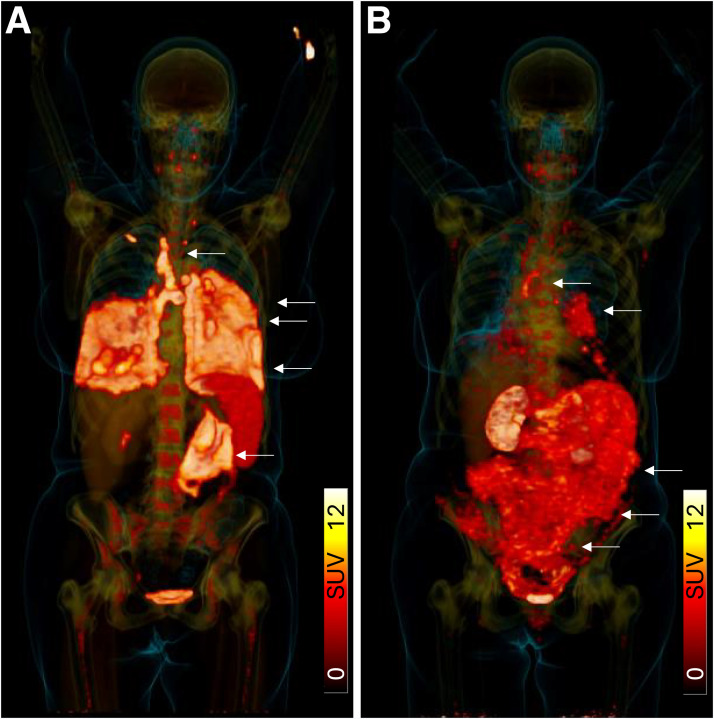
CXCR4-directed RPT with [^90^Y]Y-Pentixather holds promise particularly for salvage treatment of leukemias and lymphomas. In a 65-y-old woman with T-cell prolymphocytic leukemia and progressive disease after 4 lines of therapy, salvage treatment with [^90^Y]Y-Pentixather was offered, followed by autologous stem-cell transplantation. No further cancer drug therapy was performed. (A) Pretherapeutic [^68^Ga]Ga-Pentixafor PET/CT (3-dimensional view) identifies extensive radiotracer deposition in bilateral pulmonary manifestation sites and lymphatic/abdominal lesions. (B) Three-month follow-up [^68^Ga]Ga-Pentixafor PET/CT reveals significantly reduced tumor burden with local recurrence/progression of pleural and abdominal lesions (arrows).
